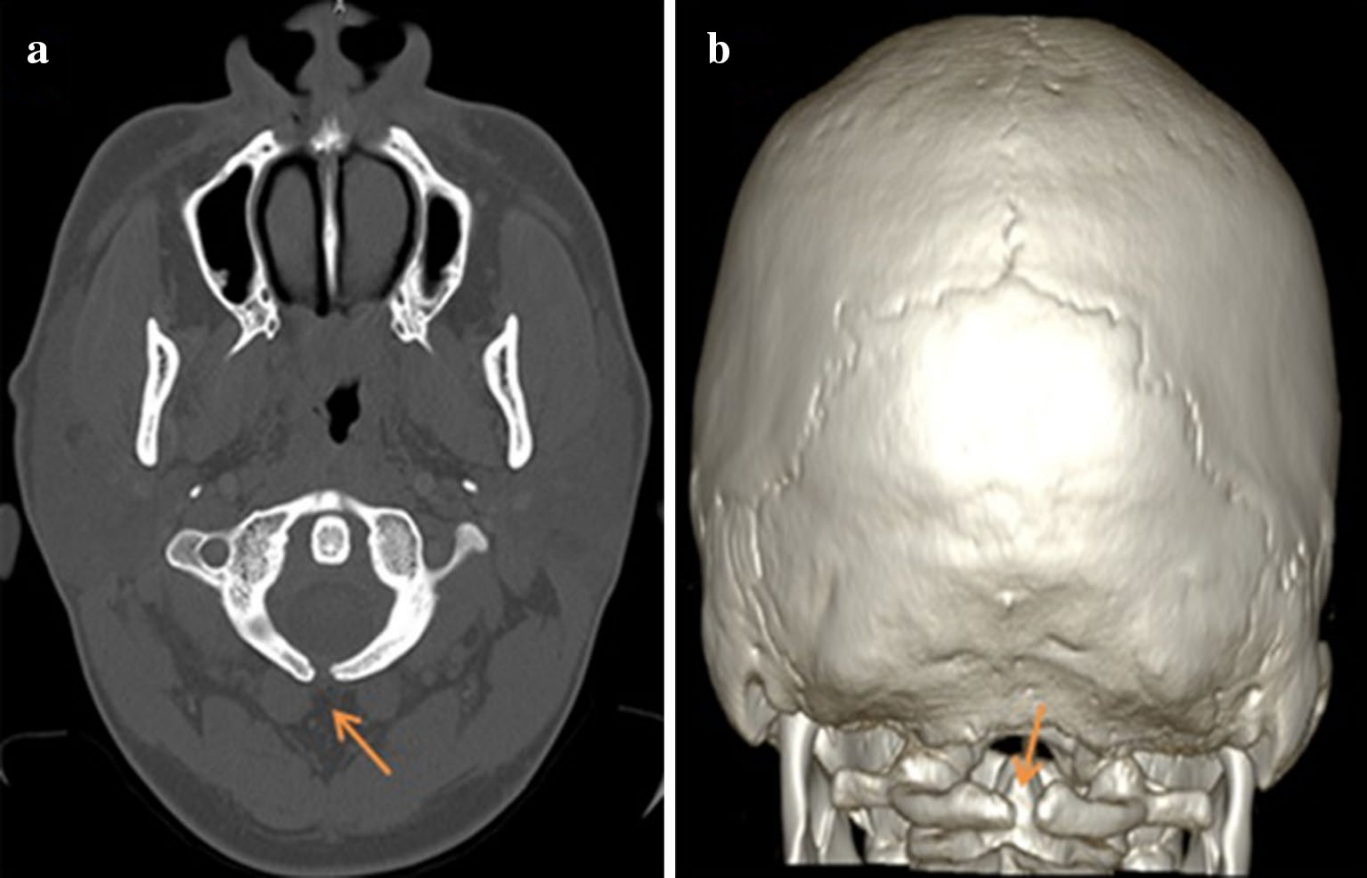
Atlas spina-bifida. Bone windows CT axial cut (a) with reconstruction VR (b) of an atlas spina-bifida occulta in a patient suffering from headache
